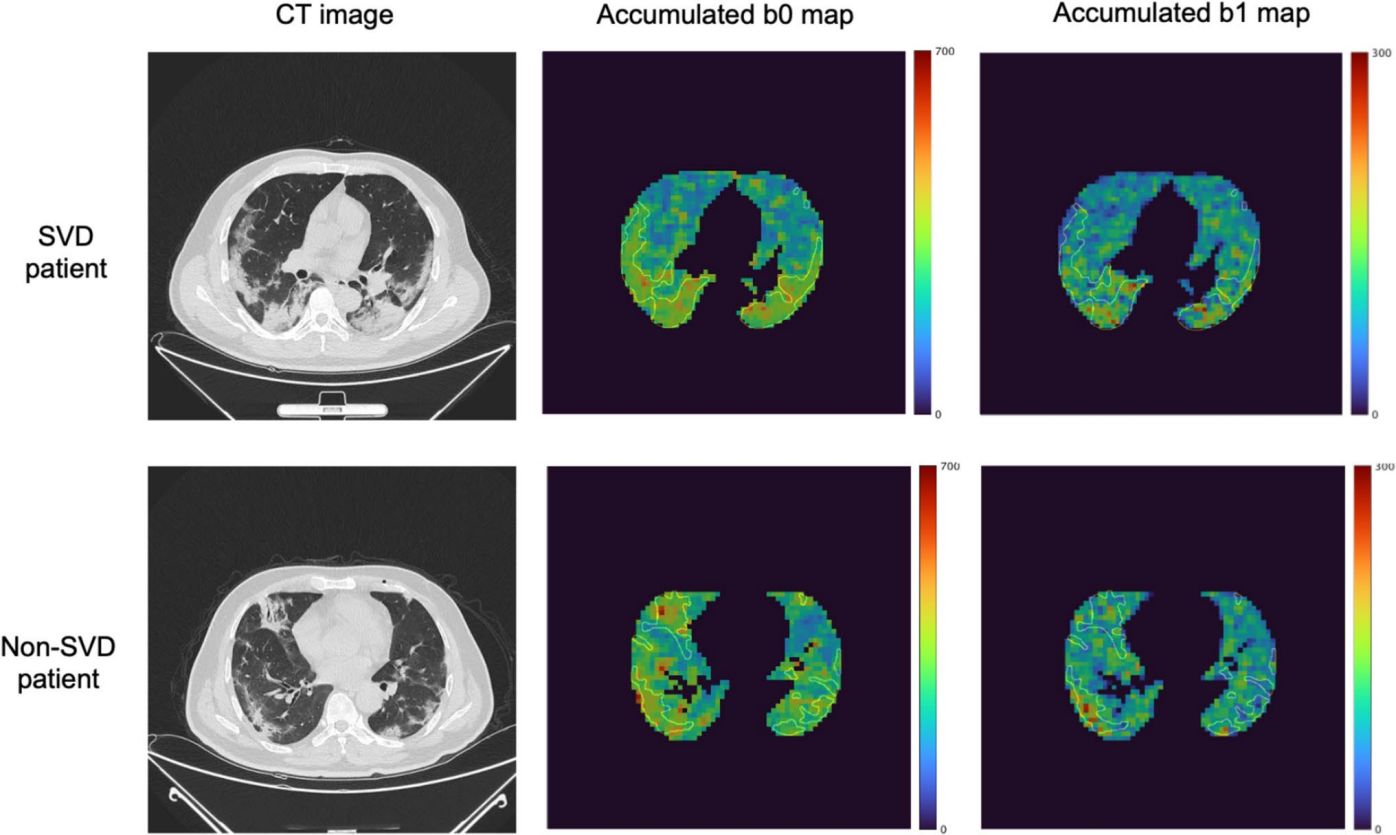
Pneumonia regions on original CT images for an SVD patient and a non-SVD patient and their corresponding accumulated BN maps with contours of the pneumonia regions. Color bars indicate the accumulated BNs from dark blue to dark red corresponding to 0 to higher numbers
